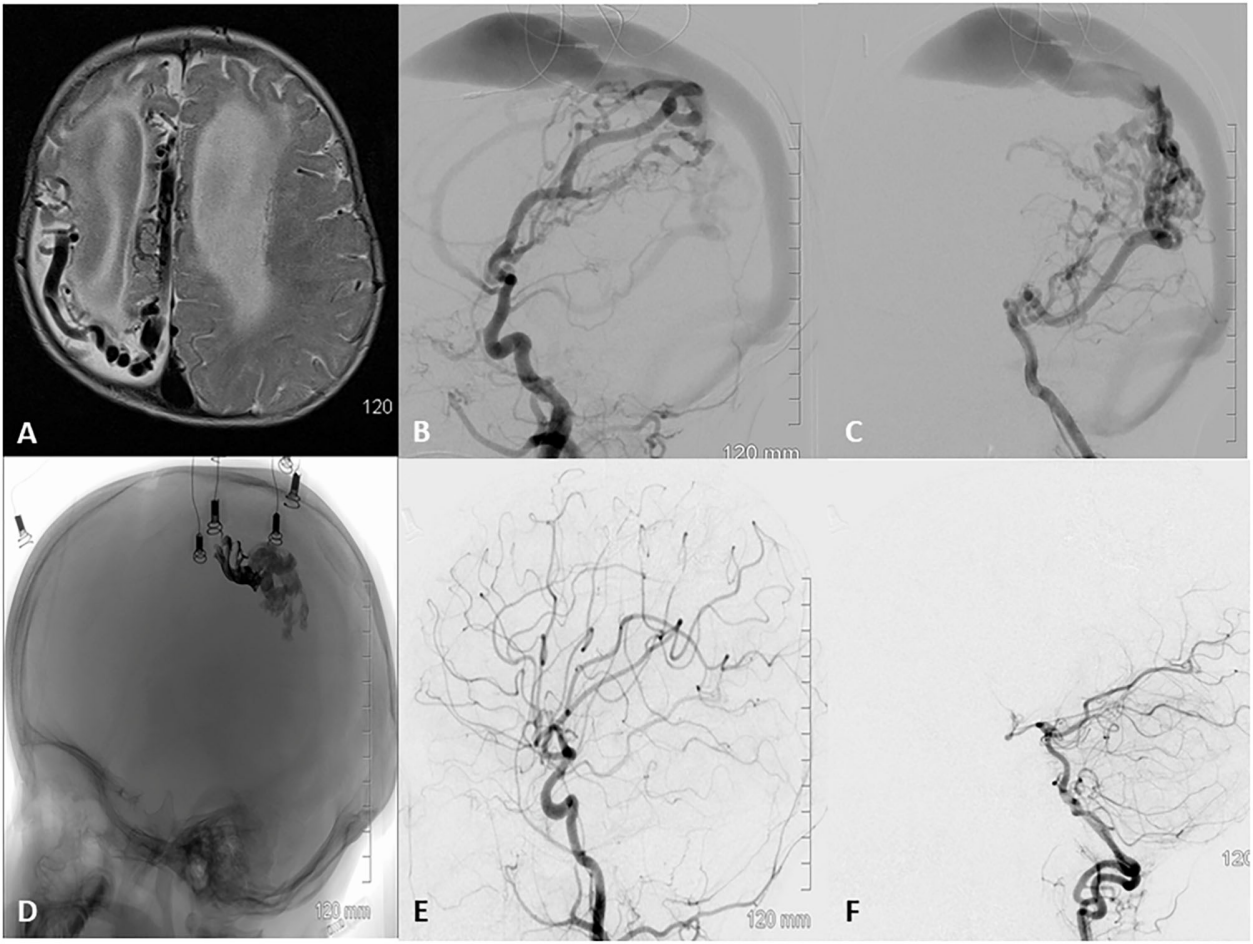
A 36 week old baby was diagnosed with a vascular malformation at 16 weeks of age. The mother noticed abnormal shape of the head with a bulge on the back of right side at 9 weeks and decreased movement of the left hand compared to the right at 16 weeks of age. The right side of the cranial vault is poorly developed, anterior fontanelle is open, full, and pulsating well. (A) MRI revealed numerous enlarged flow void in the midline and right hemisphere, with hydrocephalus and hemiatrophy on the right side of the brain. (B,C) DSA lateral view runs from the right common carotid and left vertebral revealed a pial AVF with arterial feeders arising primarily from the anterior and posterior branches of the right middle cerebral artery and the parieto-occipital branch of the posterior cerebral artery. The fistulas were multi holed and all were drained by a single enlarged venous channel that drained into the superior sagittal sinus. (D) Native lateral skull view demonstrating the final Onyx (darker anterior portion) and NBCA cast sealing several fistulae points over three staging embolization. (E,F) DSA 6 month follow up after last embolization show complete obliteration of the pial AVF with restoration of normal arterial phase.
